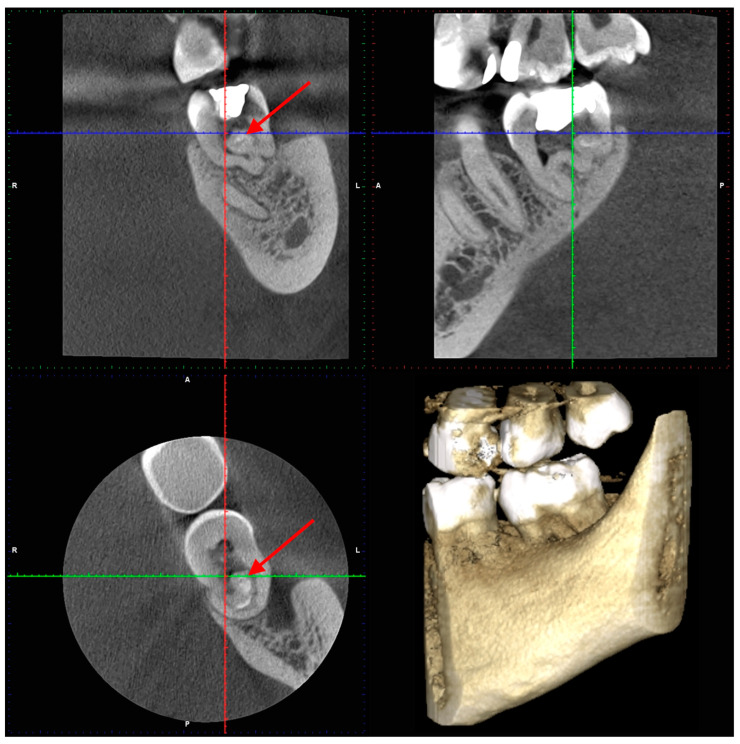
Tooth 3.7—CBCT scans (the second appointment): red arrow—the additional tooth.
